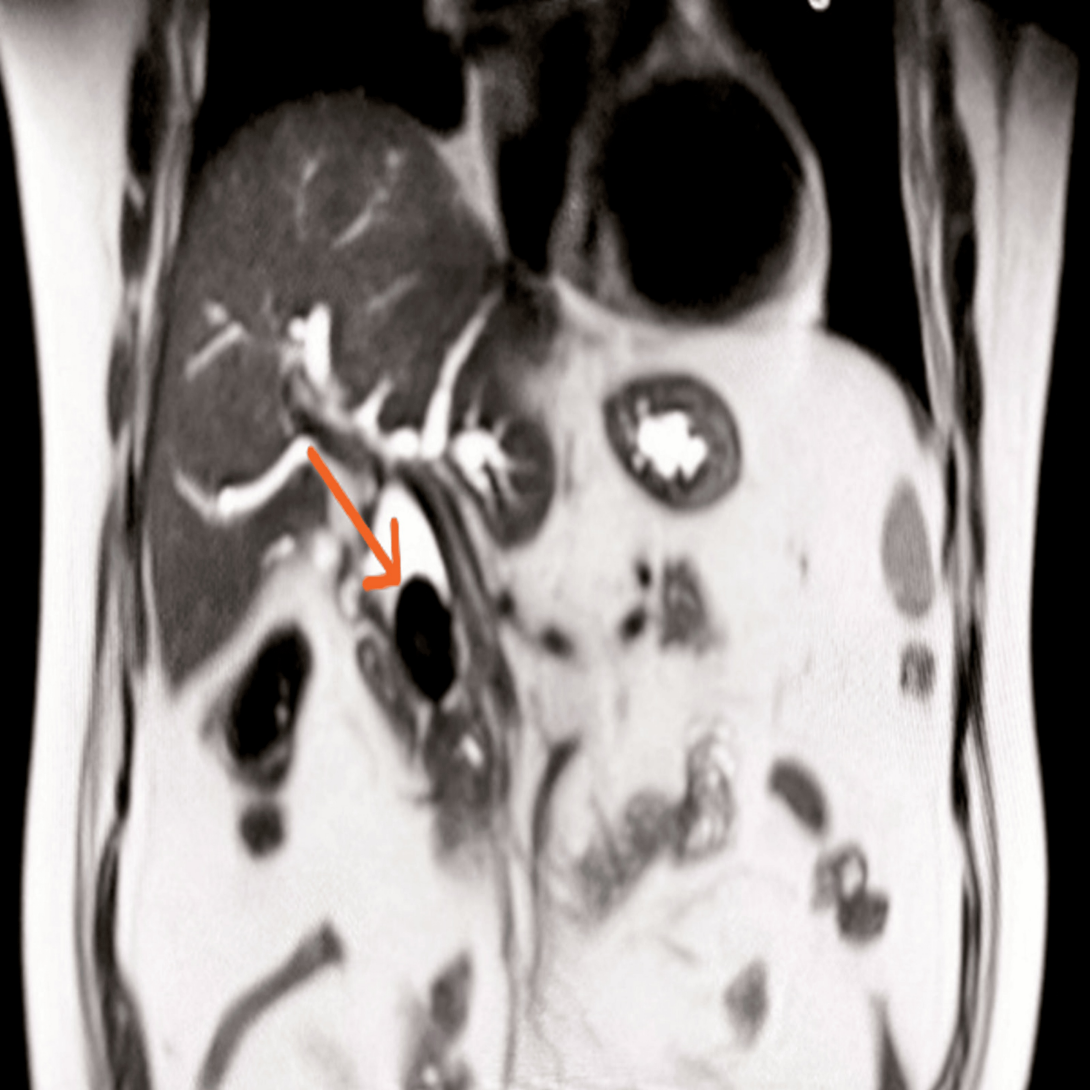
Coronal T2-weighted MRI image showing an intraductal filling defect in the region of the mid common bile duct (CBD), suggestive of an intraductal calculus
